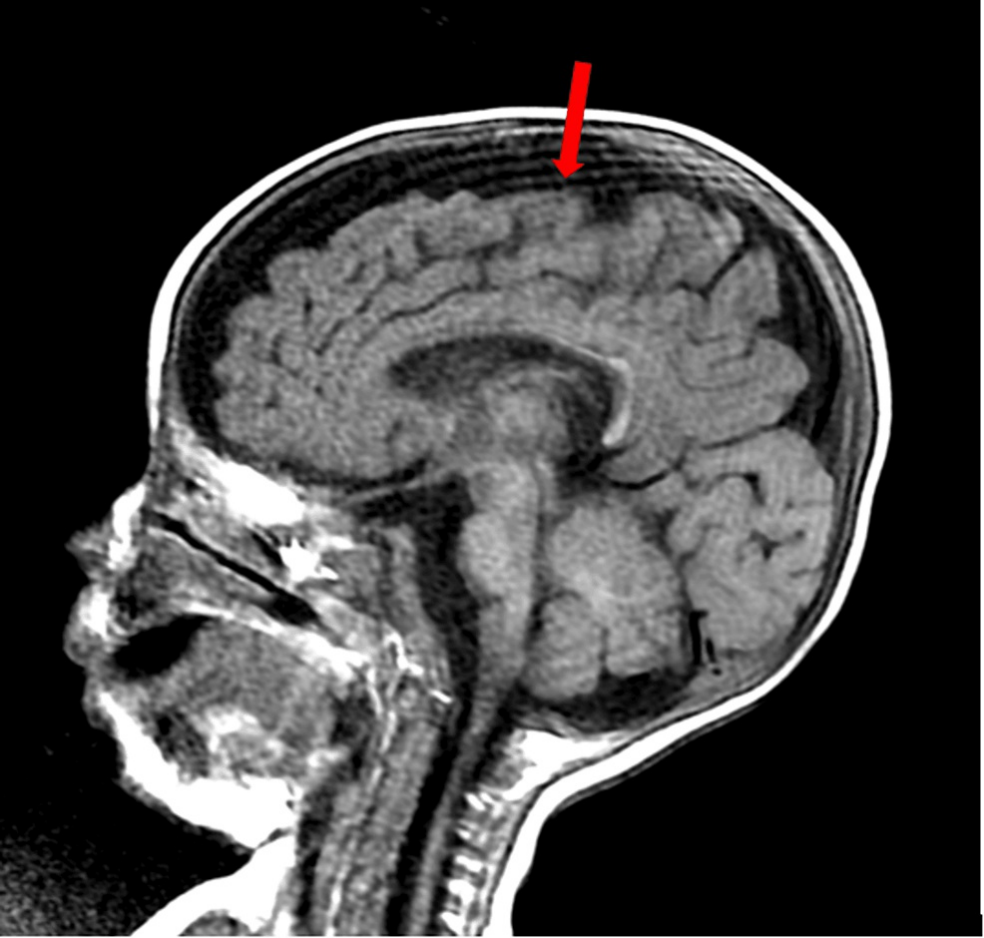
Sagittal slice of a brain MRI scan showing widening of the cortical sulci in relation to cortical atrophy
